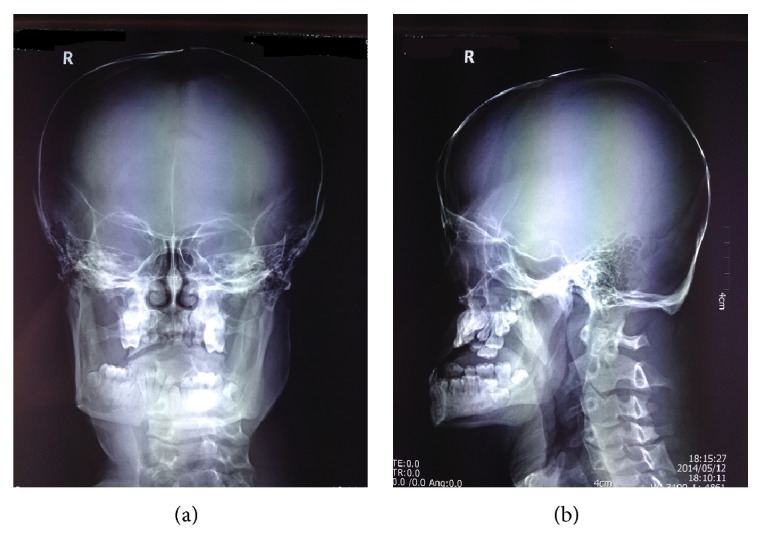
Head X-ray images. (a) Front head X-ray images presented that his teeth were sparse and irregular. Part of the permanent teeth was absent on the hypoplastic upper alveolar ridge; (b) lateral head X-ray images show that the upper alveolar ridge was depressed and lower alveolar ridge was prominent.
